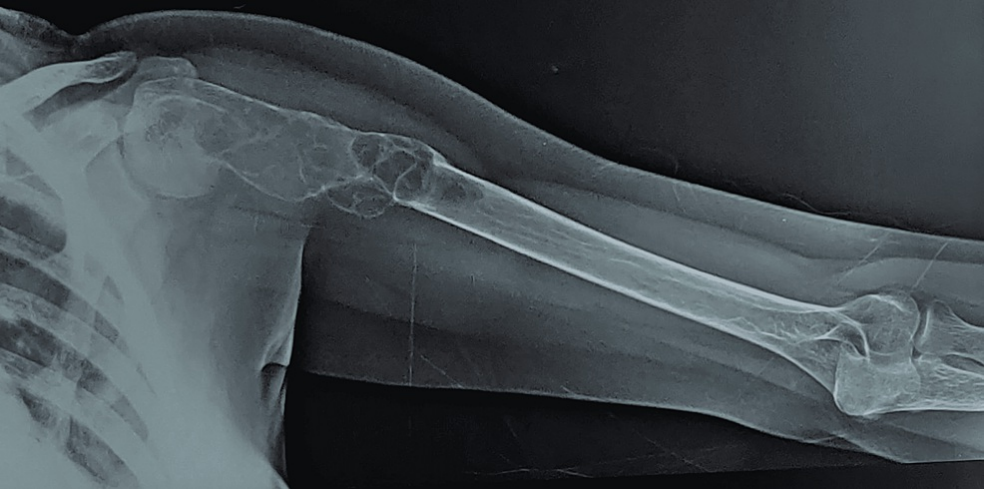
X-ray showing fracture in the left arm mimicking fibrous dysplasia
